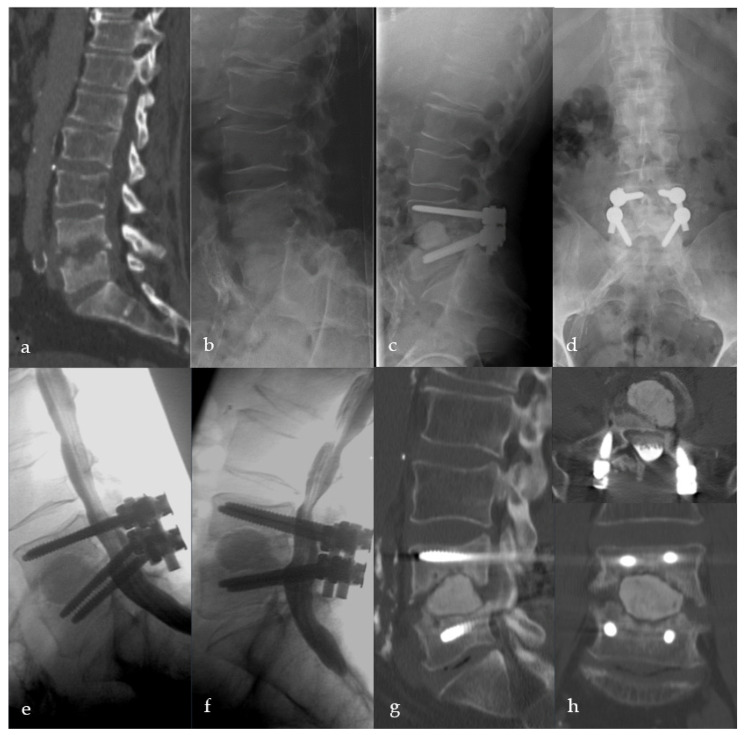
Sixty-two-year-old male patient with severe destructive spondylodiscitis L4/5. Preoperative CT-Scheme and lateral X-ray (a,b). Three-month follow-up radiograph (c,d). X-ray and CT scan one year after surgery showing anterior, intervertebral pseudarthrosis with stable posterior implants (e–h).
